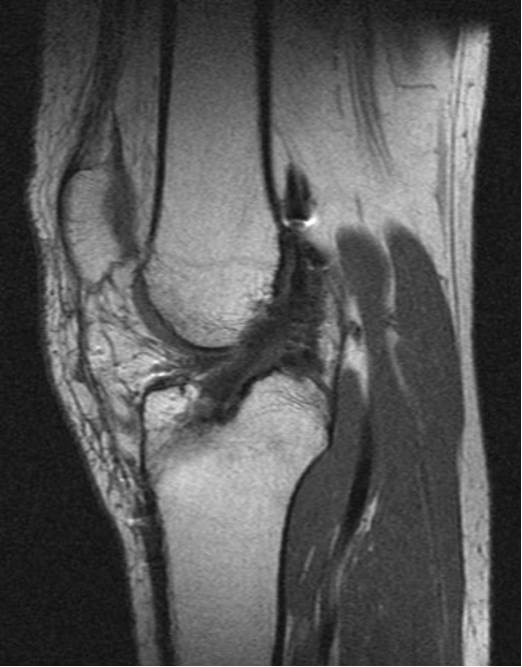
Post operative MRI scan showing intact ACL (sagittal view)
